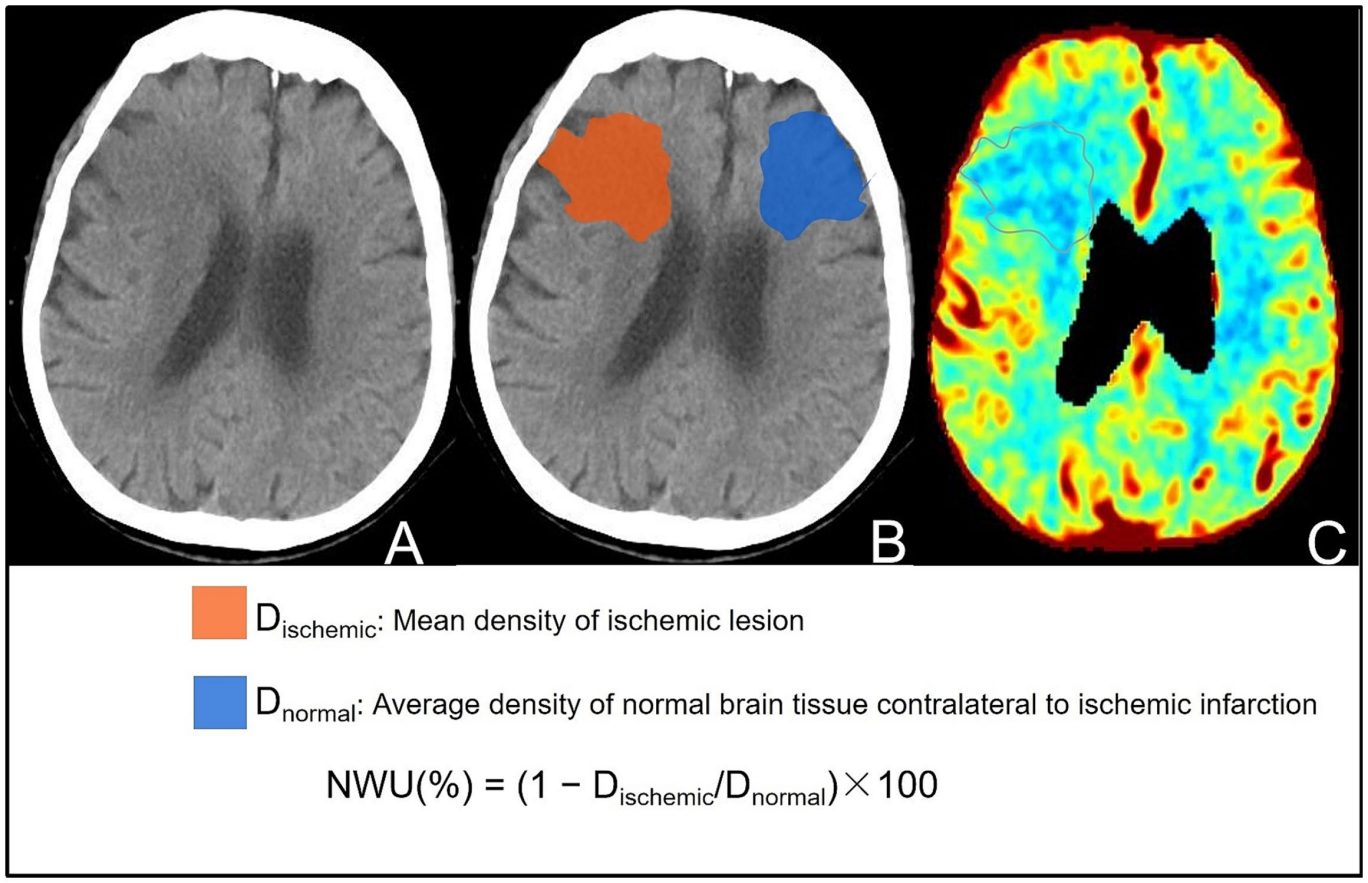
Visual representation of the steps for calculating NWU on the baseline NCCT images of the same patient. (A) NCCT image showing patchy slightly low attenuation near the lateral ventricle of the right frontal lobe. (B) Segmented lesion area (red: ischemic lesion area; blue: opposite normal brain tissue). The NWU was calculated based on the average CT value of the segmented area, and the calculation formula is shown in the figure. (C) CBV map verifying the accuracy of the ischemic partition, showing consistency between reduced CBV and the partitioned area.
